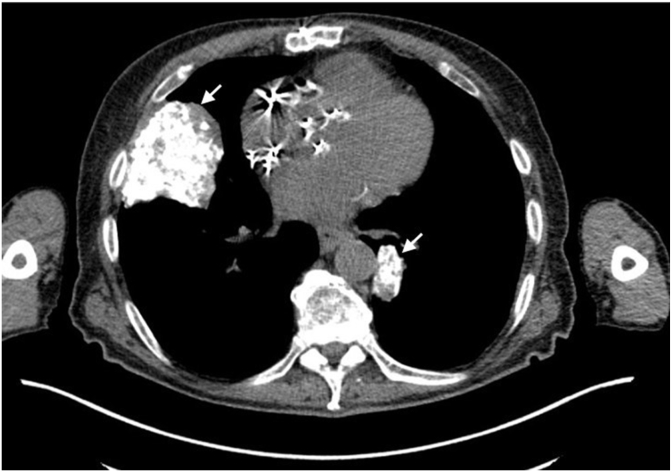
CT Chest confirming calcification in pulmonary nodules (arrows).
